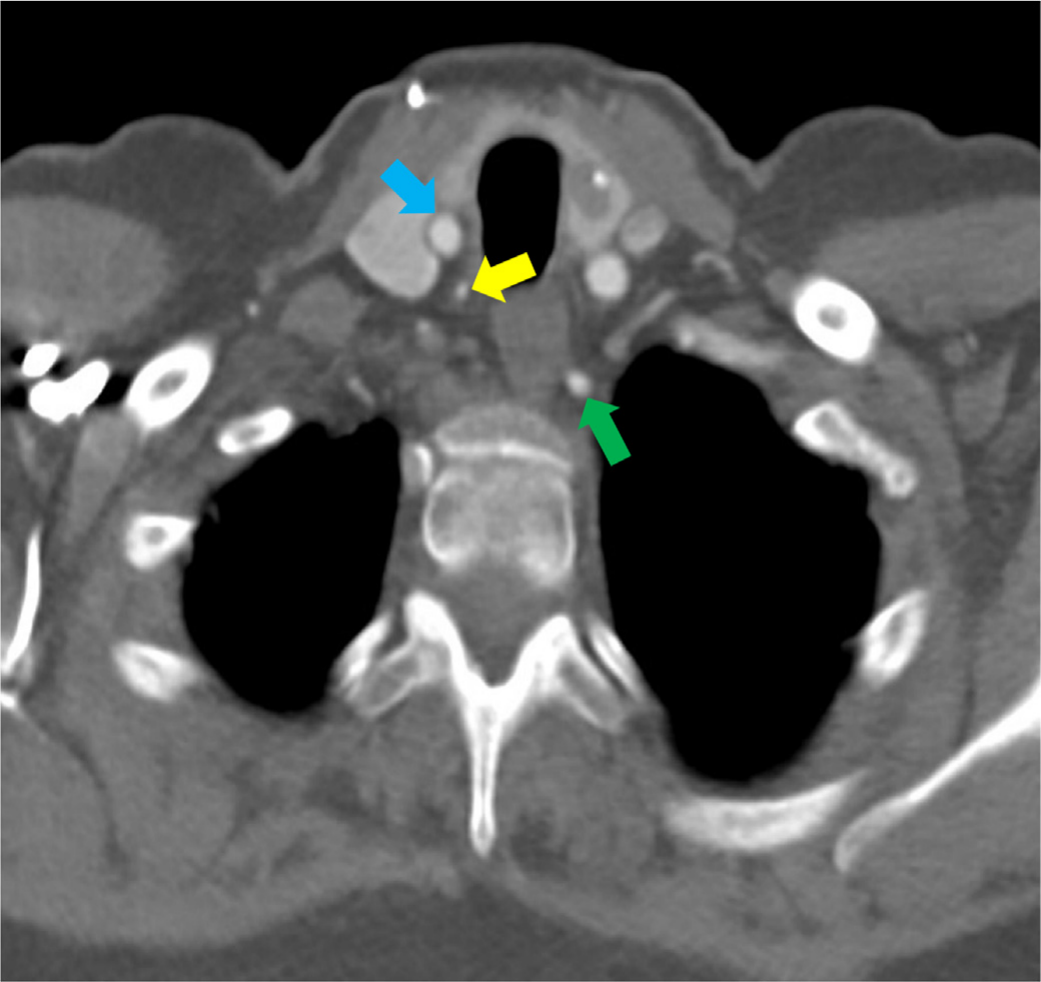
Course of the right vertebral artery at the thoracic inlet: Axial CT near the thoracic inlet shows the right vertebral artery (yellow arrow) next to the right common carotid artery (blue arrow). Also visible is the left vertebral artery (green arrow).
